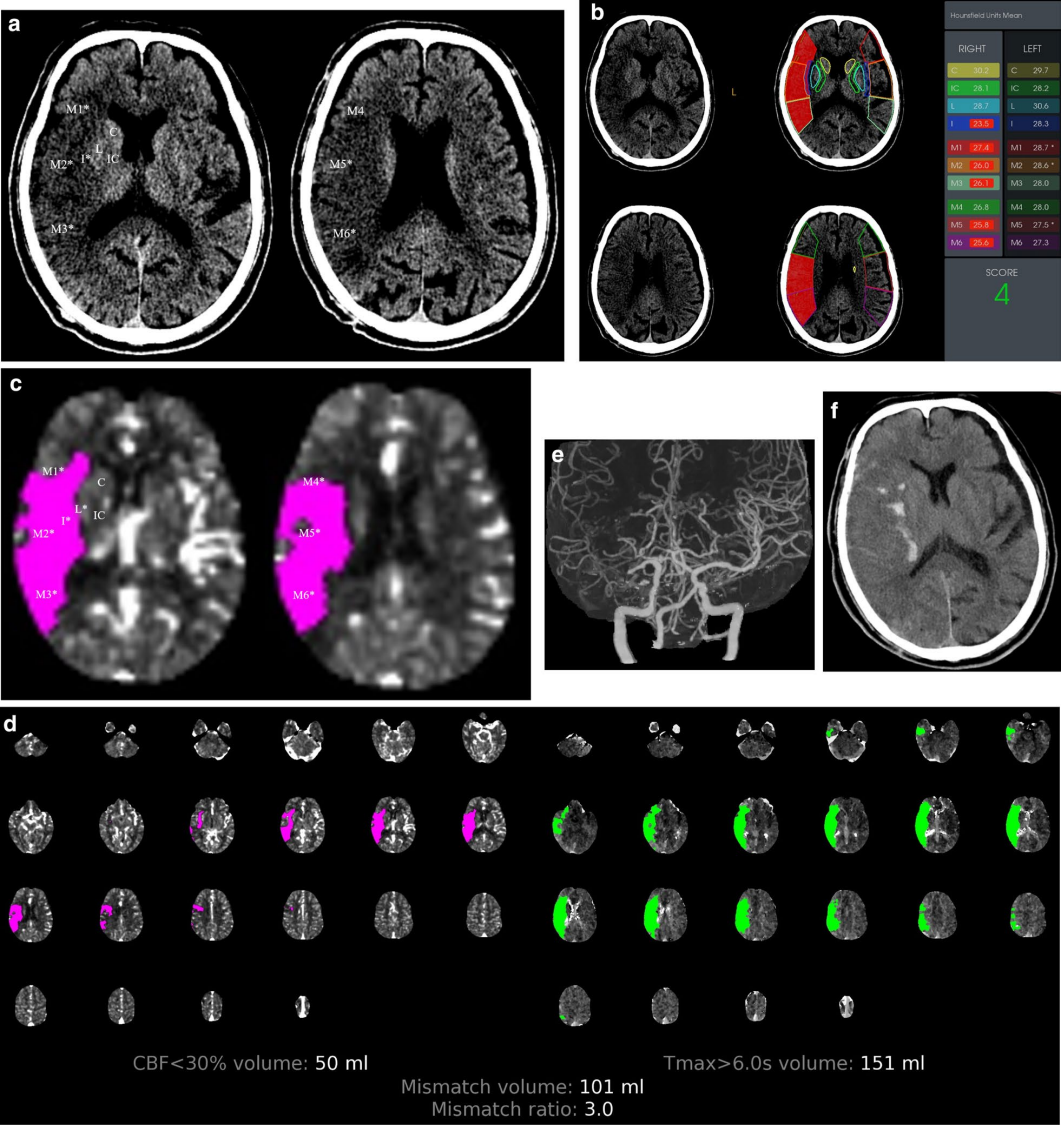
A 75-year-old male patient was sent to the emergency department 8 h after the onset of symptoms with a baseline NIHSS score of 22. The NCCT-, Auto- and CTP-ASPECTS were 4, 4, and 2 points (a–c), respectively. CT perfusion revealed an infarct core of 50 ml, hypoperfusion of 151 ml, mismatch volume of 101 ml, and mismatch ratio of 3.0 (d). The maximum intensity projection showed that the proximal M1 segment of the right middle cerebral artery was occluded (e). The patient successfully underwent mechanical thrombectomy 65 min after admission. A review of the NCCT 14 h after revascularization revealed a large infarction of the right temporal lobe with intracranial hemorrhage (f). The patient had a poor clinical outcome at the 90-day follow-up. * indicates the regions of the early ischemic changes of the 10 defined middle cerebral artery (MCA) vascular territories. C = caudate head, I = insula, IC = internal capsule, L = lentiform nucleus, M1 = frontal operculum, M2 = anterior temporal lobe, M3 = posterior temporal lobe, M4 = anterior MCA, M5 = lateral MCA, M6 = posterior MCA
